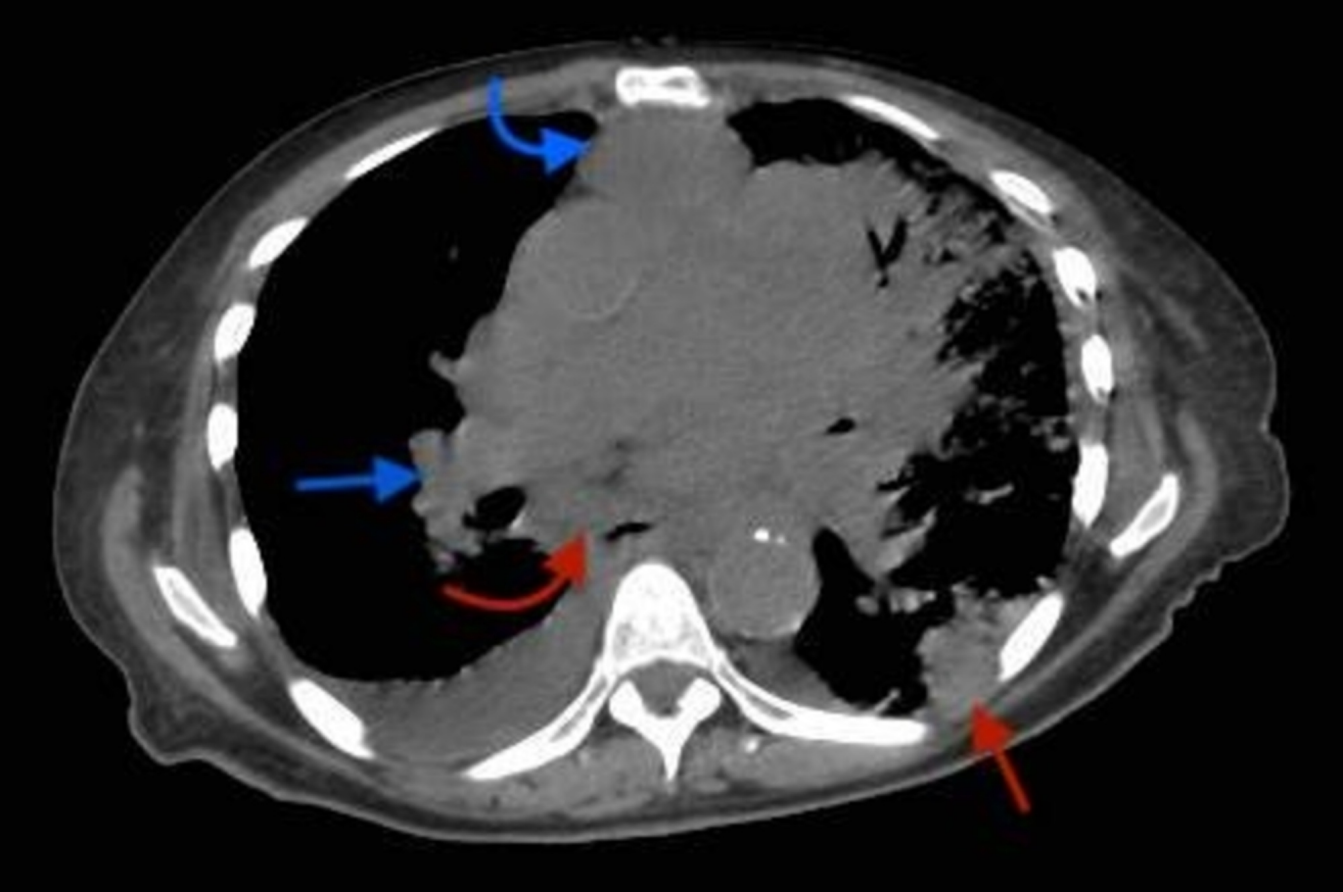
Axial CECT (contrast-enhanced computed tomography) scan at T6 level showing metastatic focus in basal part of right upper lobe with right hilar lymph nodal enlargement (straight blue arrow), enlarged retrosternal/anterior mediastinal lymph node (curved blue arrow), enlarged para-esophageal lymph node (curved red arrow), metastatic pleural involvement (straight red arrow).
